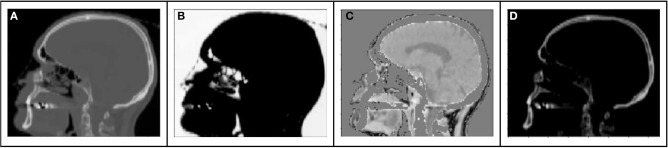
Example sagittal CT image with tissue windows: (A) original CT image (B) air window (C) tissue window, (D) bone window.
